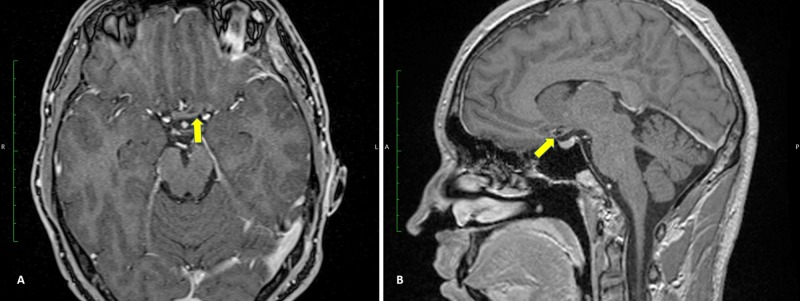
Postoperative (A) axial and (B) sagittal MRI.
